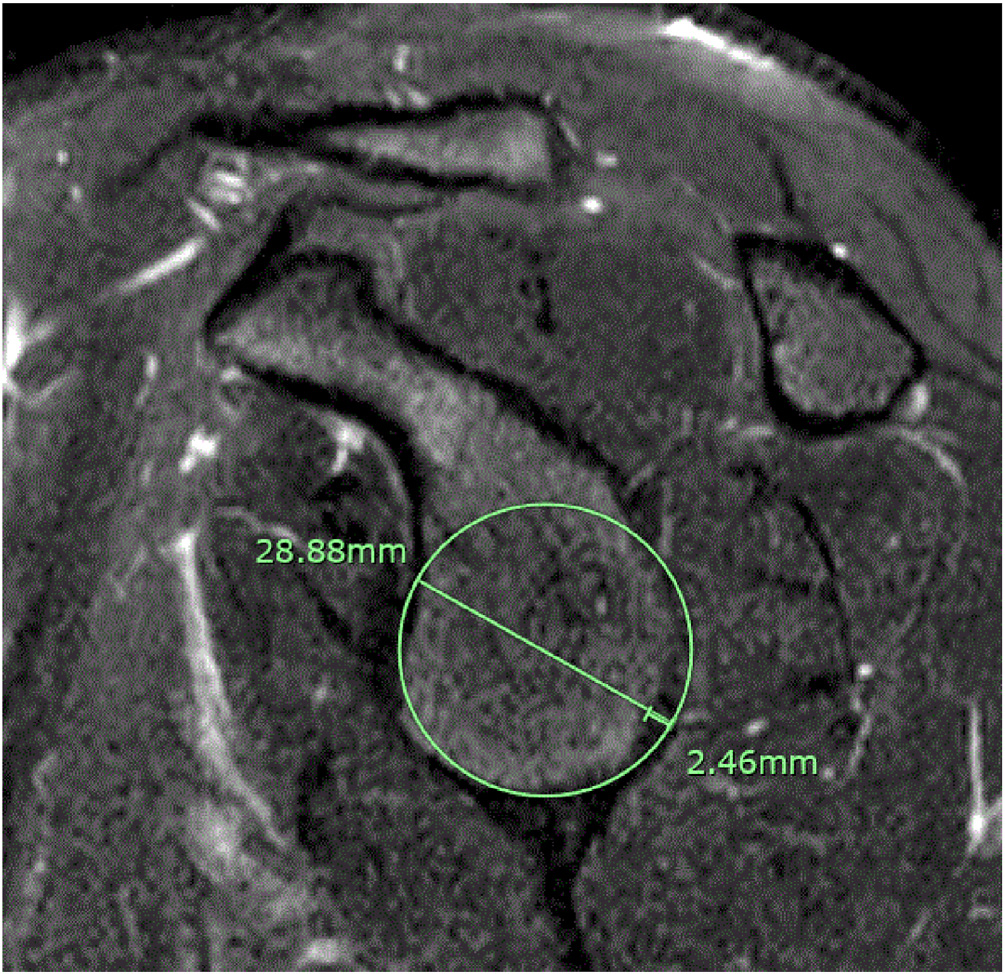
MRI sagittal view at the glenoid face of the left shoulder in the plane of the glenoid. (MRI, magnetic resonance imaging.)
